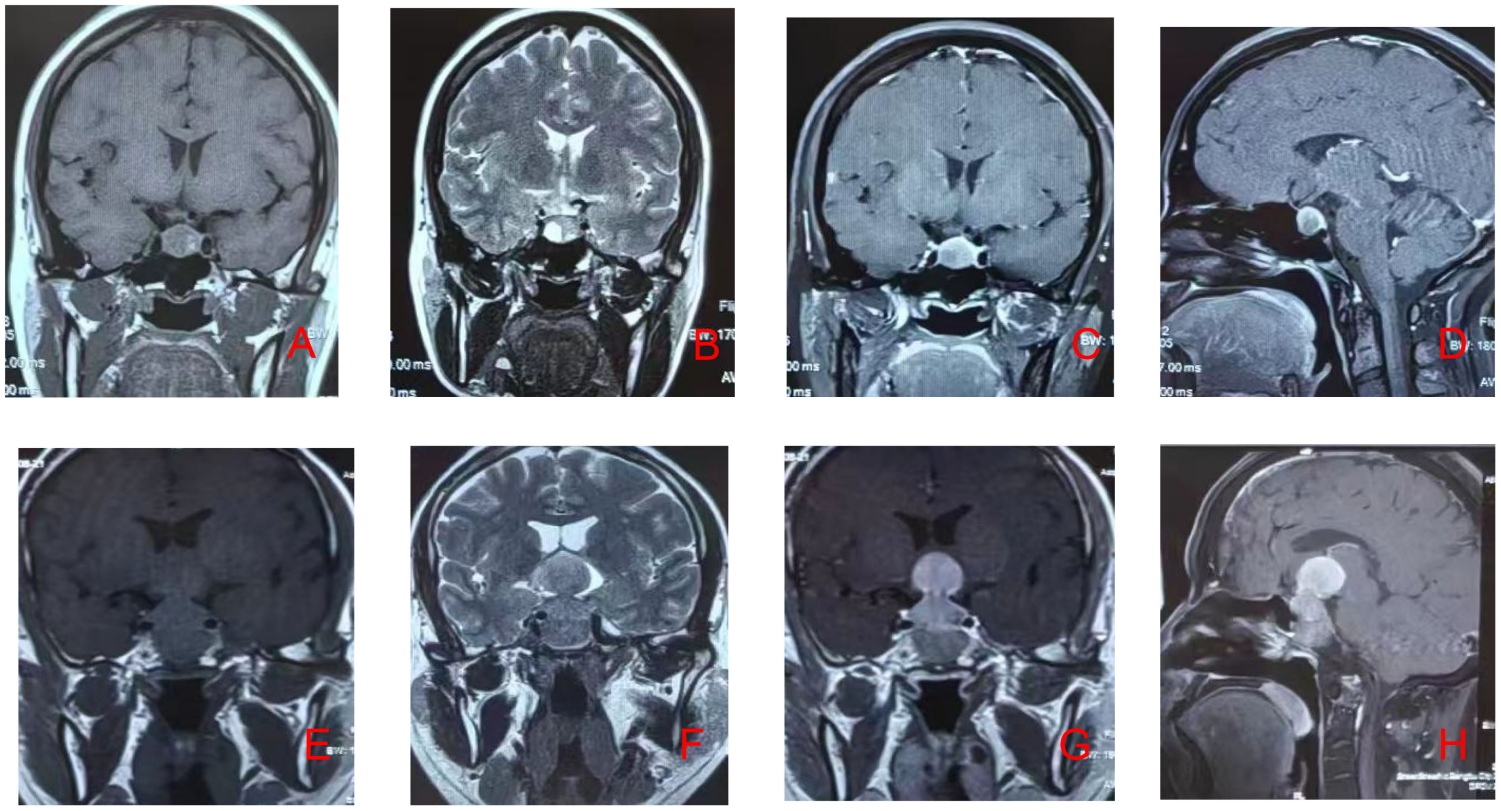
Preoperative MRI images of non-invasive and invasive pituitary adenomas. Panels (A–D) show MRI scans of a patient with a non-invasive pituitary adenoma, while panels (E–H) display MRI scans of another patient with an invasive pituitary adenoma.
